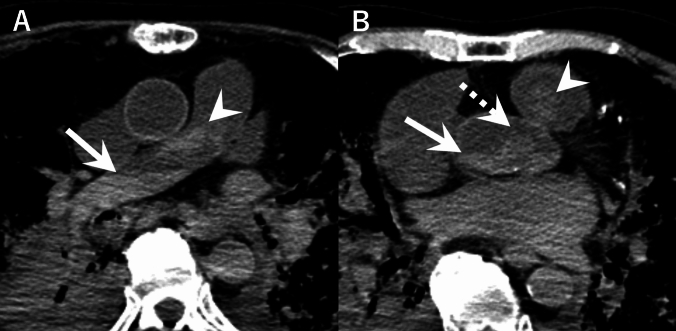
Example of uncertain differentiation between a blood clot and gravitational sedimentation. A 79-year-old man with interstitial pneumonia. A Axial postmortem CT showing a mixed pattern of gravitational sedimentation (arrow) and clot-like high-density area (arrowhead) in the pulmonary artery. B Axial postmortem CT showing gravitational sedimentation (arrow) and clot-like high-density areas in the ascending aorta (dotted arrow) and pulmonary artery (arrowhead). CT was performed 482 min after death
